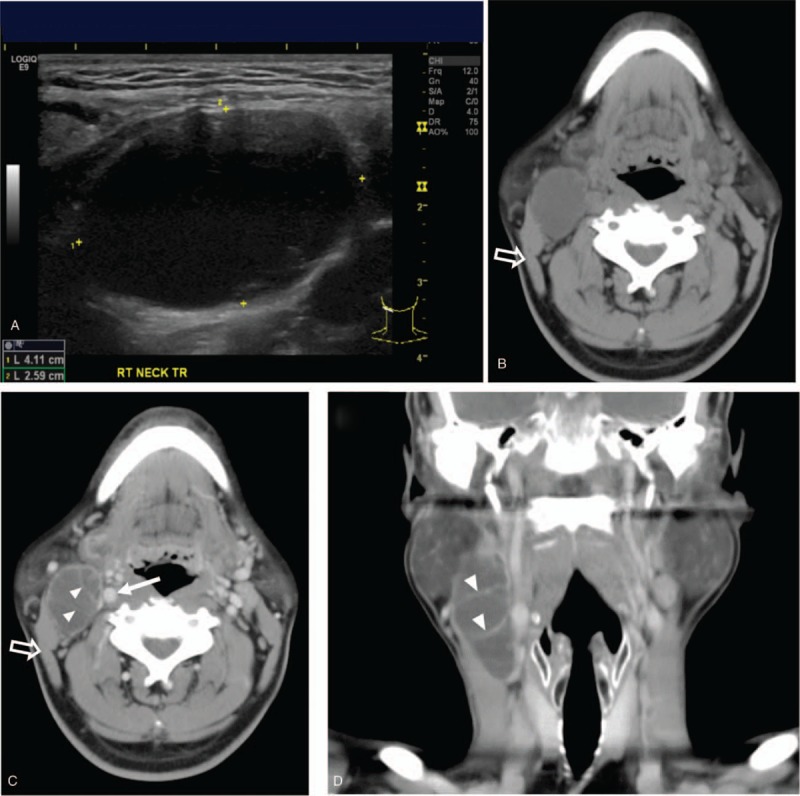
Preoperative image findings. (A) Neck sonography shows a cystic mass in the submandibular region measuring 4.11 cm. (B) Axial non-contrast computed tomography (CT) image, (C and D) axial and coronal contrast-enhanced CT images, respectively, show a cystic mass measuring 6.4 × 4.0 × 2.9 cm with septations (arrowheads) anterior/medial to the sternocleidomastoid muscle (outline arrows) and lateral to the carotid artery (white arrow).
